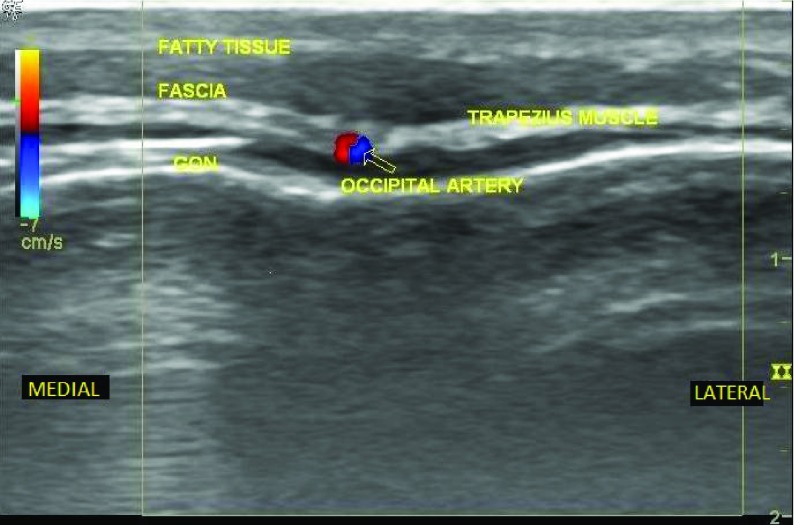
Patient 1; Ultrasound Image of the Greater Occipital Nerve in Relation to the Occipital Artery, Fatty Tissue, Fascia, and the Trapezius Muscle. GON = Greater occipital nerve
